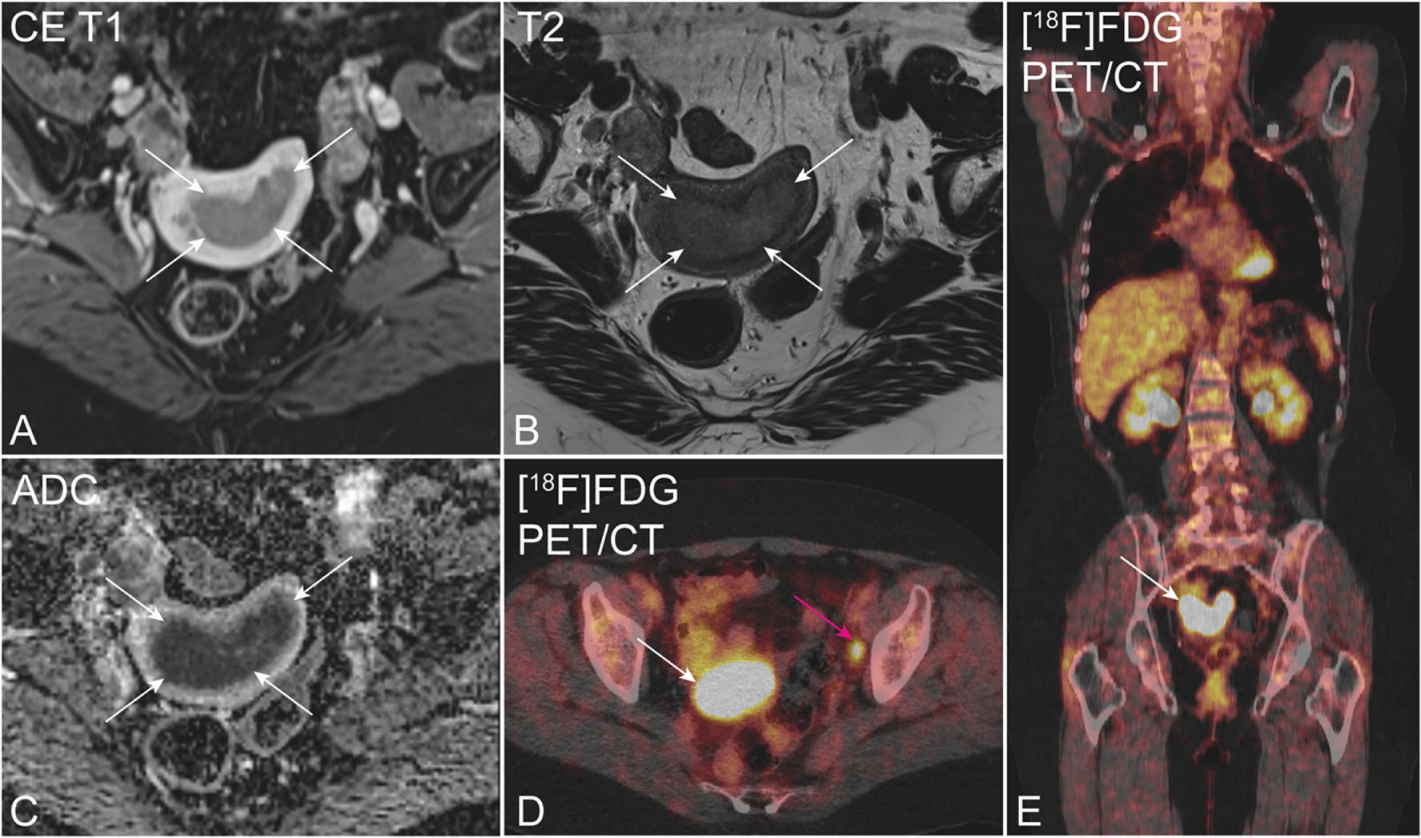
Preoperative pelvic MRI (A–C) and [^18^F]FDG PET/CT (D, E) in a 72-year-old patient with endometrial carcinoma, FIGO stage IIIC (endometrioid, grade G1). Axial oblique contrast-enhanced T1-weighted (A) and T2-weighted MRI (B) depicts an arcuately shaped uterine tumor (white arrows), exhibiting restricted diffusion at apparent diffusion coefficient map (C). Axial (D) and coronal (E) [^18^F]FDG PET/CT shows elevated [^18^F]FDG uptake both in the primary tumor (white arrow) and in a left iliac lymph node (cerise arrow)
